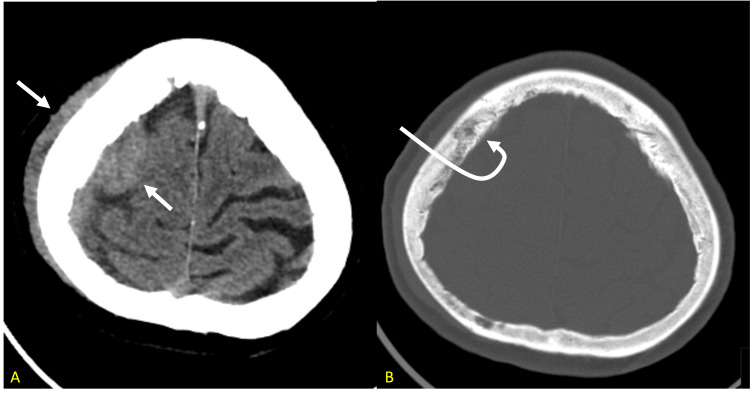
a. CT head (soft tissue window) demonstrating a right frontal extra-axial lesion and an extracranial soft tissue component within the scalp. b. The bone window suggests subtle permeative bone marrow infiltration of the right parietal bone.
